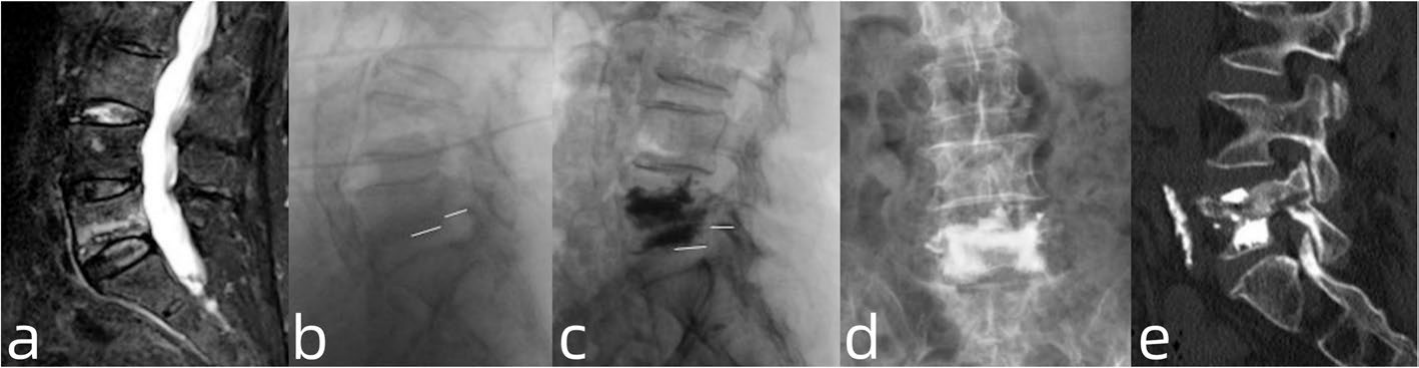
a Sagittal view of T2-weighted MRI showing the fracture of L5 IEP and decreased posterior vertebral body height; b, c: Comparison of pre- and post-operative radiography regarding the height between IEP and inferior margin of pedicle; d: Postoperative radiography showing satisfactory distribution of bone cements; e: The postoperative sagittal CT showing no bone cement leakage
